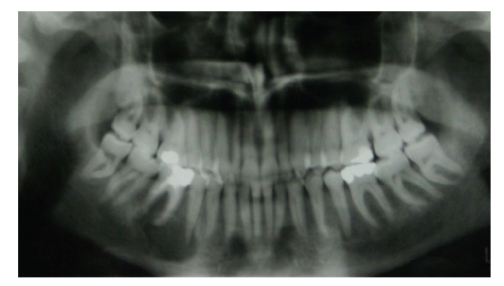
(December 12, 2000) X-ray of the maxilla that highlighted a bony rarefaction area.
